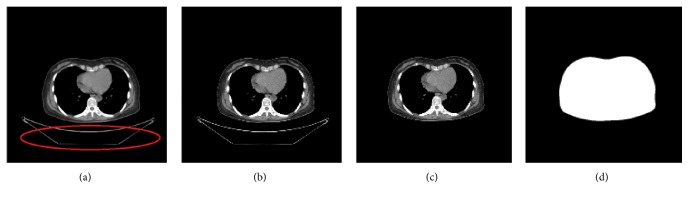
An example of CT preprocessing results. (a) The source CT image. (b) The normalization result. (c) The final preprocessing result (segmented result). (d) The feature image.
